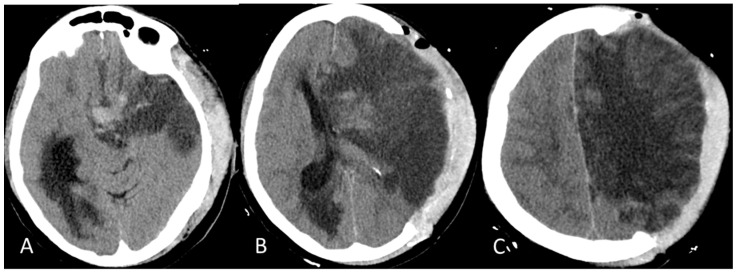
CT Brain on last day. (A) Transtentorial uncal herniation compressing the midbrain bridge from left to right; blood secretion in the nucleocapsular and subnucleocapsular regions; (B) significant compression on the III and on the left SV, with significant shift to the right and hydrocephalus of the right SV with signs of transependymal CSF transudation; and (C) clear and diffuse cortico-subcortical hypodensity of ischemic-edematous significance in the anterior cerebral artery (ACA) and middle cerebral artery (MCA) vascularization territories, from “malignant” cerebral infarction; the artery territories partly preserved posterior cerebral (posterior occipitotemporal) results of left fronto-parietal decompressive craniotomy with brain herniation (“brain fungus”).
